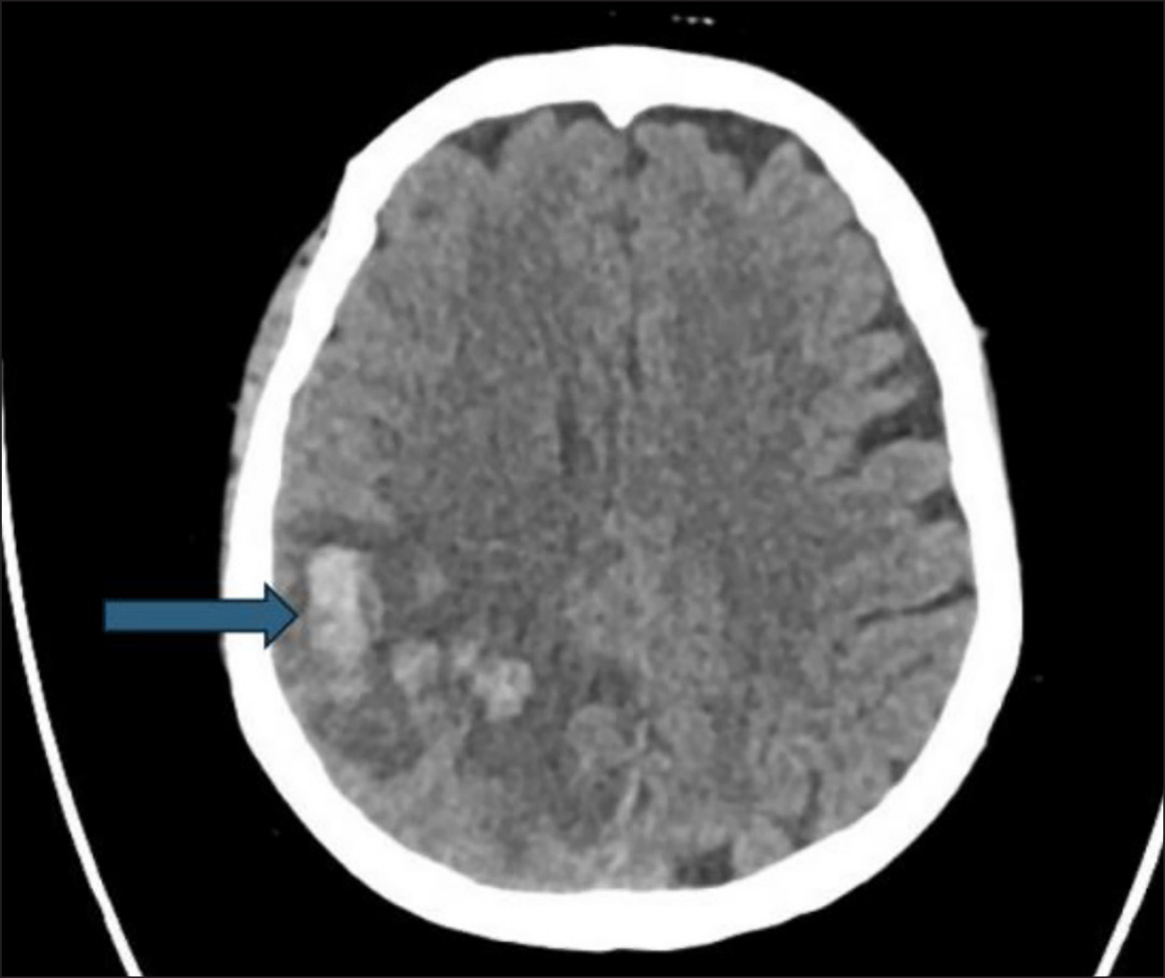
CT of the head showing multiple hyperdense lesions in the right parietal regions, consistent with hemorrhagic infarcts.
